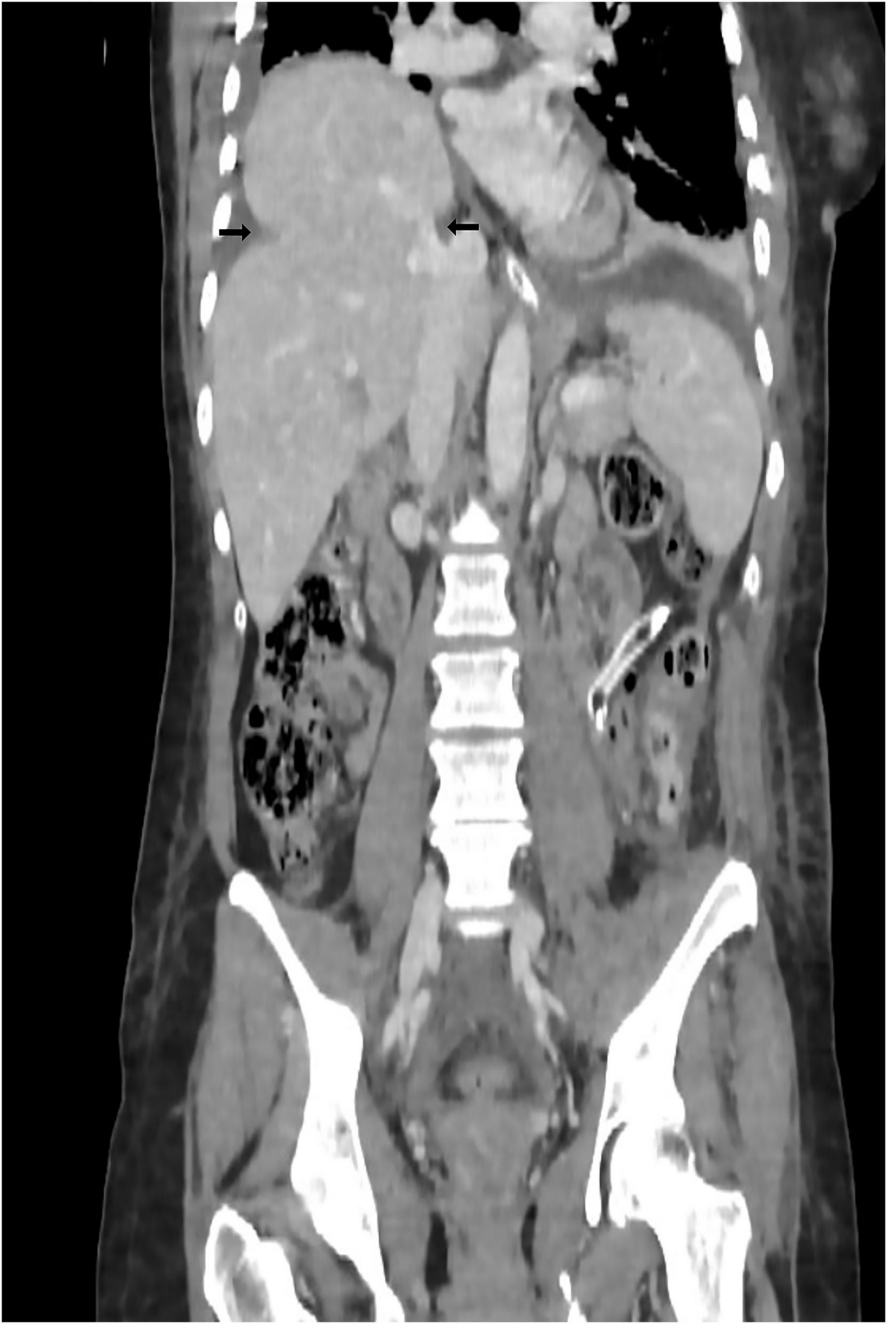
Fourth postoperative day Thorax-Abdomen contrast-enhanced CT that showed the raising of the right liver dome and collar sign (black arrows) with herniation of the VII and VIII liver segments.
